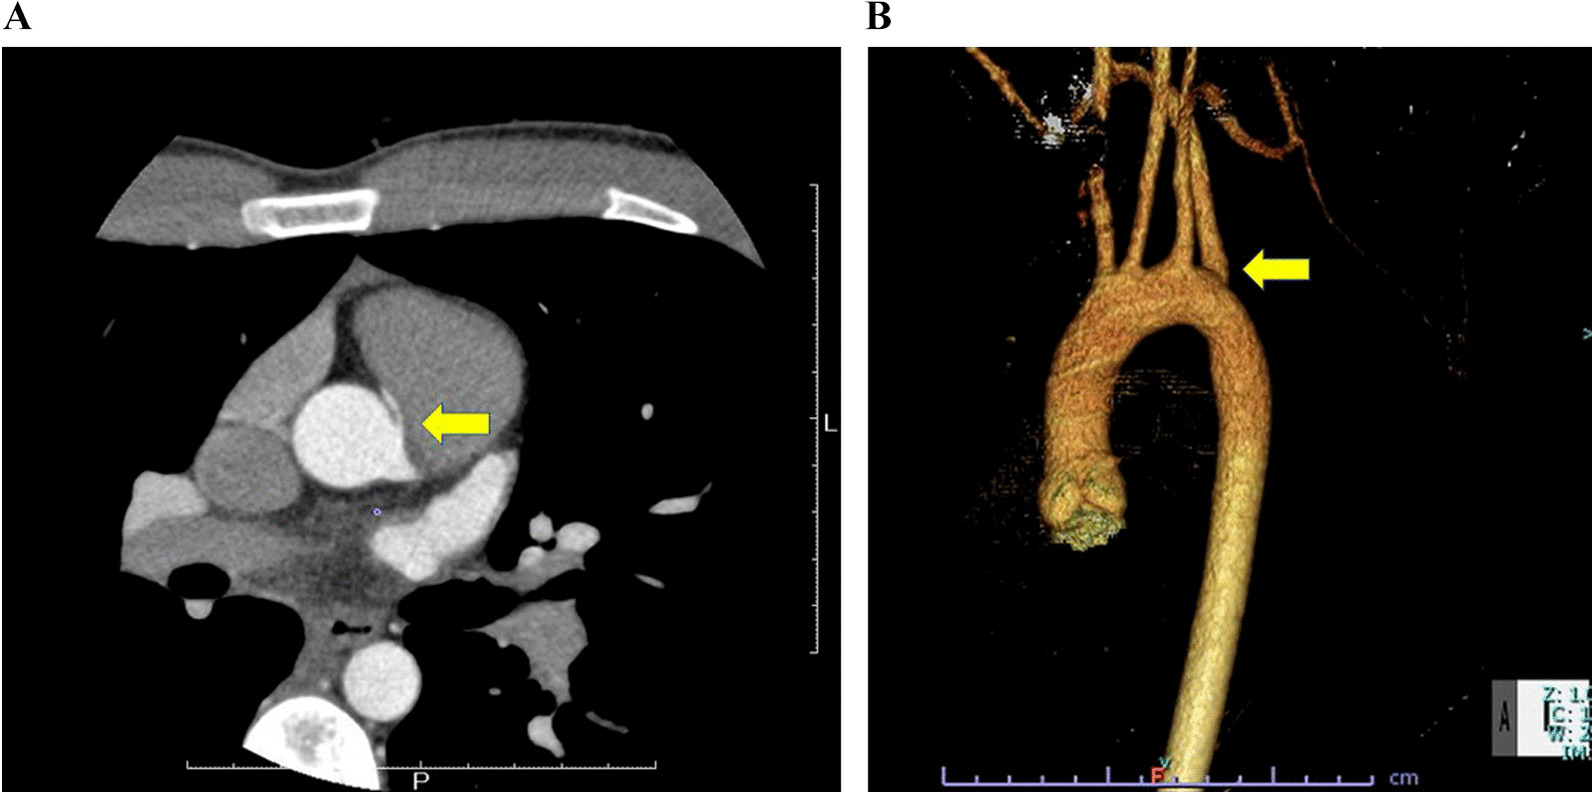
A Coronary CT (computed tomography) revealed anomalous aortic origin of a coronary artery. B Three-dimensional CT revealed an aberrant right subclavian artery
